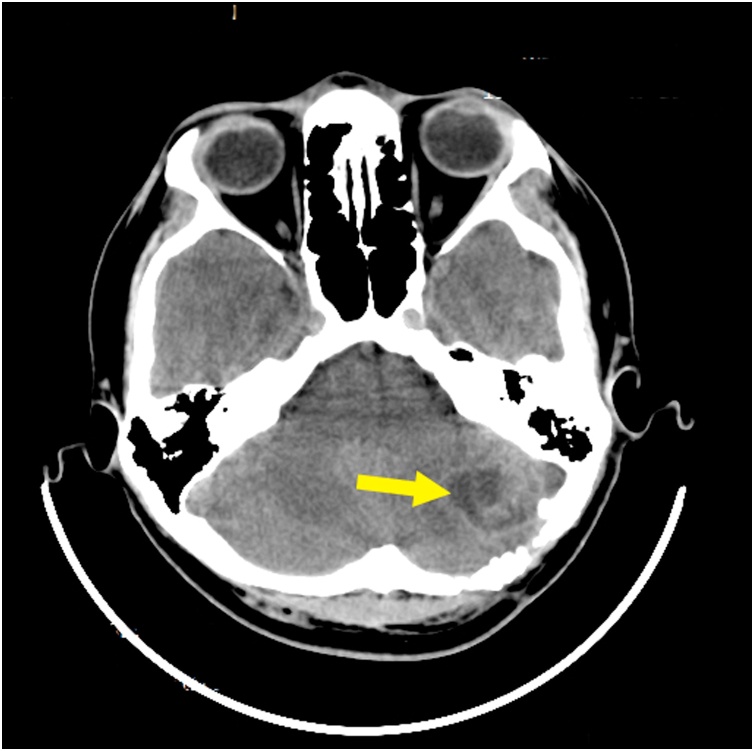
Three month postoperative enhanced head CT images showed the brain abscess was relatively consistent with its previous size, shape, and location.
